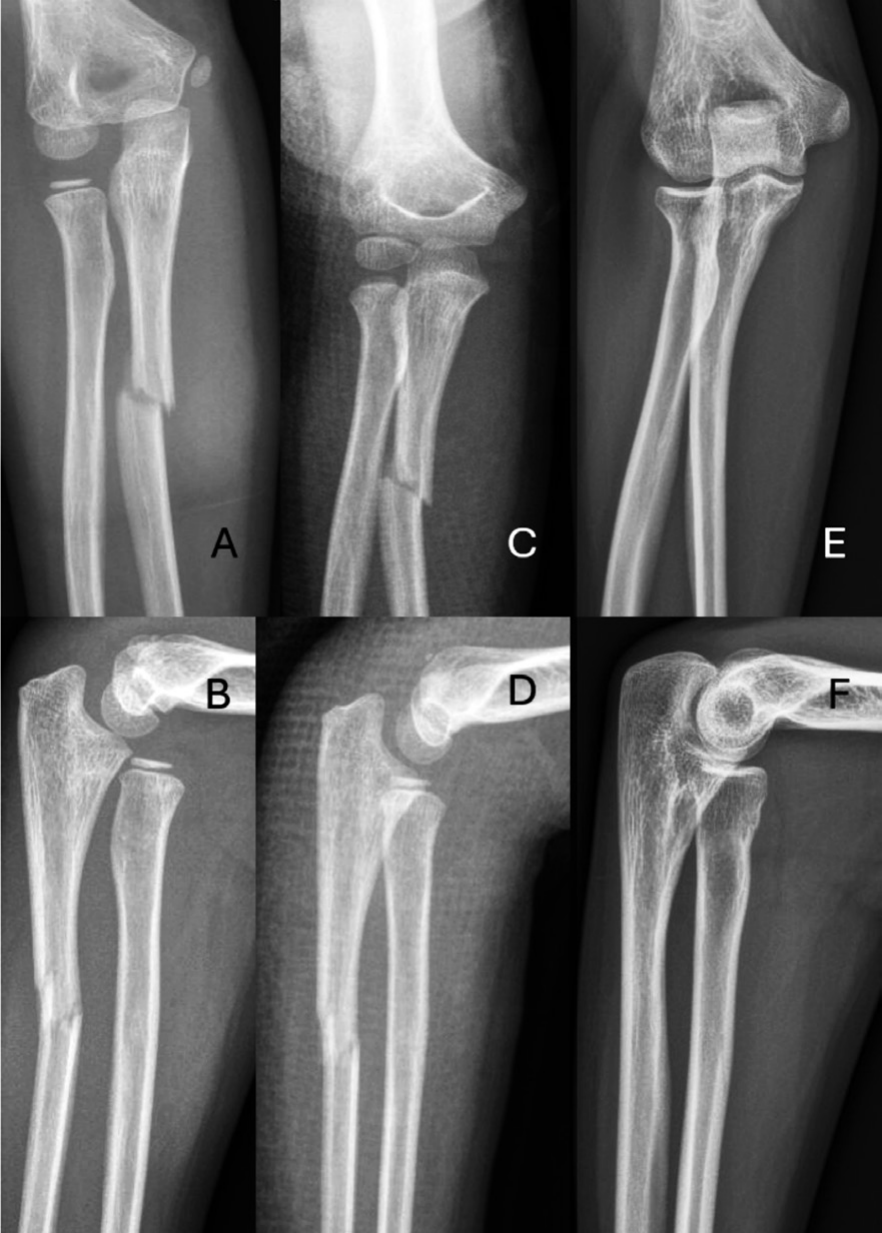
A 7-year old girl with Monteggia fracture Bado type I (A, B) treated with closed reduction and cast (C, D). Injury had healed fully radiographically and functionally 8 years after injury (E, F).
